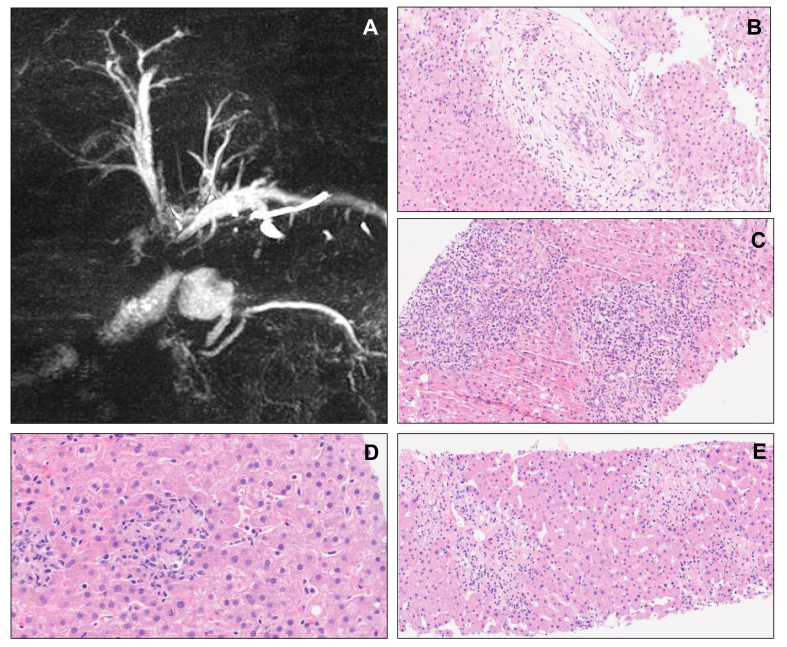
Morphologic assessment and liver biopsies. (A) Magnetic resonance imaging showing sclerosing cholangitis. (B) Lymphocytic cholangitis (HES 20×). (C) Neutrophilic cholangitis (HES 20×). (D) Granulomatous hepatitis (HES 40×). (E) Pericentrolobular necrosis and endothelitis (HES 20×). HES, Hemalun-Eosine-Safran coloration.
